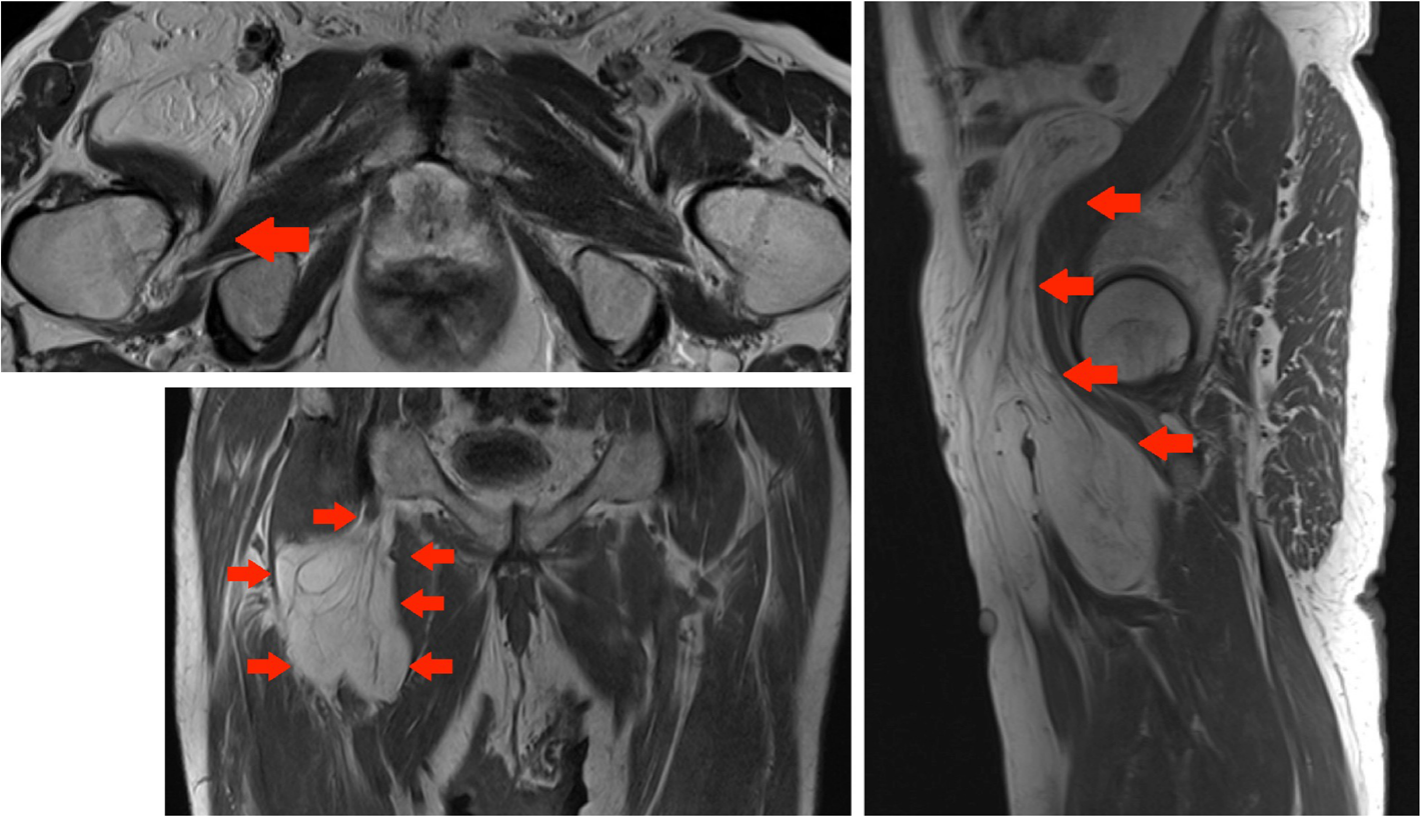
Top left panel: Axial T2-weighted MR image of the pelvis: a large lipomatous tumour arising from the right iliopsoas compartment is seen to extend to the iliopsoas insertion onto the lesser trochanter (red arrow). Bottom left panel: Coronal T1-weighted MR image of the pelvis: large lipomatous mass in the right iliopsoas compartment (red arrows). Internal septations and ‘haziness’ of the fat suggest a more aggressive lesion. Right panel: Sagittal T1-weighted image of the pelvis: Lesion of fat signal intensity continuous with the iliopsoas
